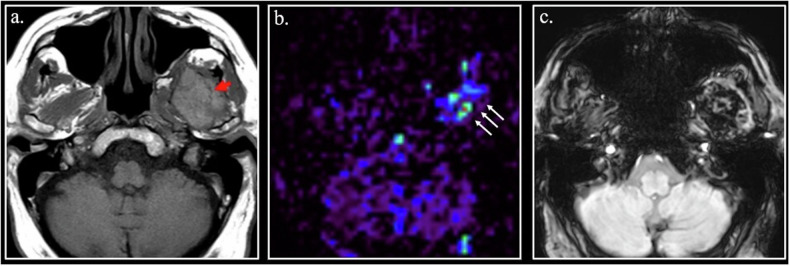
Unenhanced FSE T1-weighted scan (a) shows a left masticatory space soft tissue metastasis by clear cell renal adenocarcinoma, resected 10 years before. Intralesional flow-void vessels can be detected into the hypervascularized lesion (red arrow). A pseudo-continuous Arterial Spin Labeling (pCASL) scan (b) confirms the high vascularization of the lesions (white arrows). Susceptibility Weighted Imaging (SWI) sequence (c) demonstrates intralesional hypointense, slow-flow vessels containing deoxiHemoglobin
